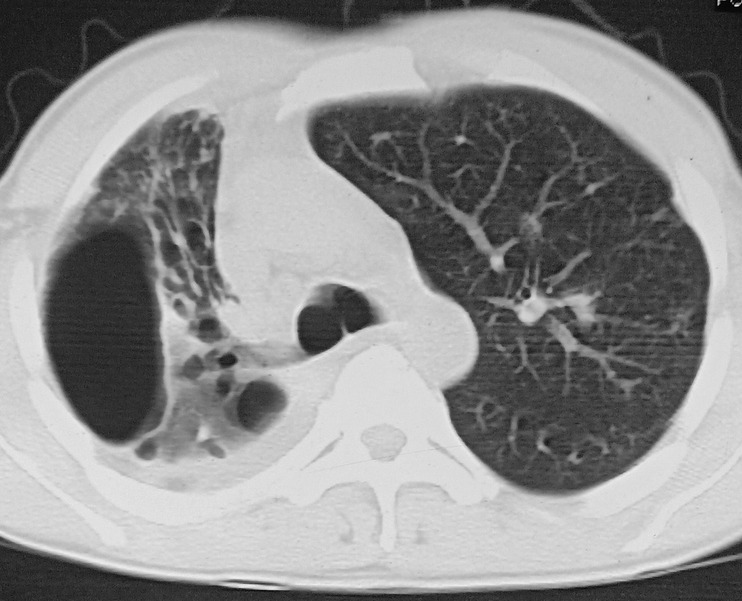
Computed tomography (CT) of the chest showing cavity and fibro‐bronchiectatic changes in the right upper lobe.
